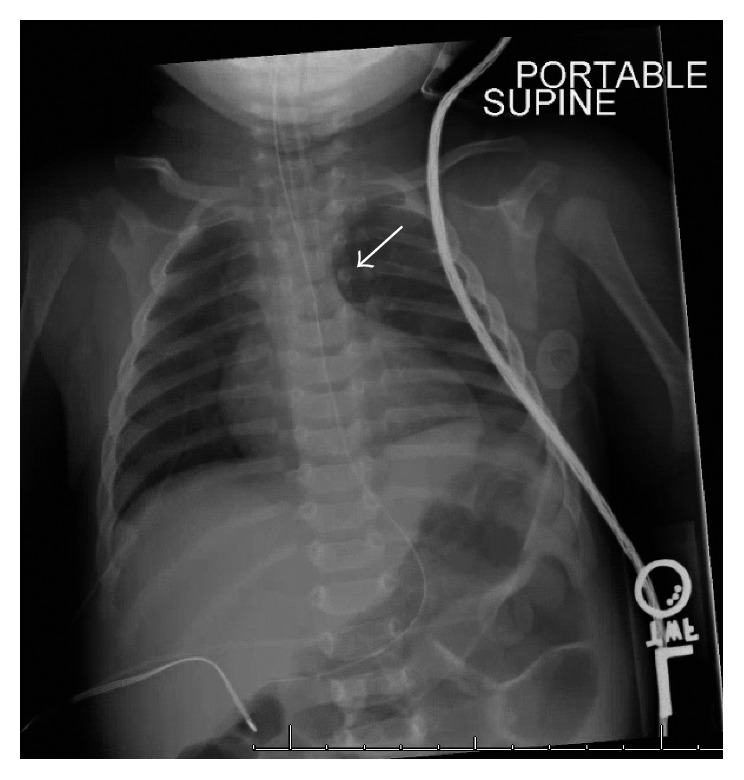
Portable X-ray which shows an absence of the aortic knob (arrow) and inability to visualize the descending aorta, suggestive of a right-sided aortic arch.
